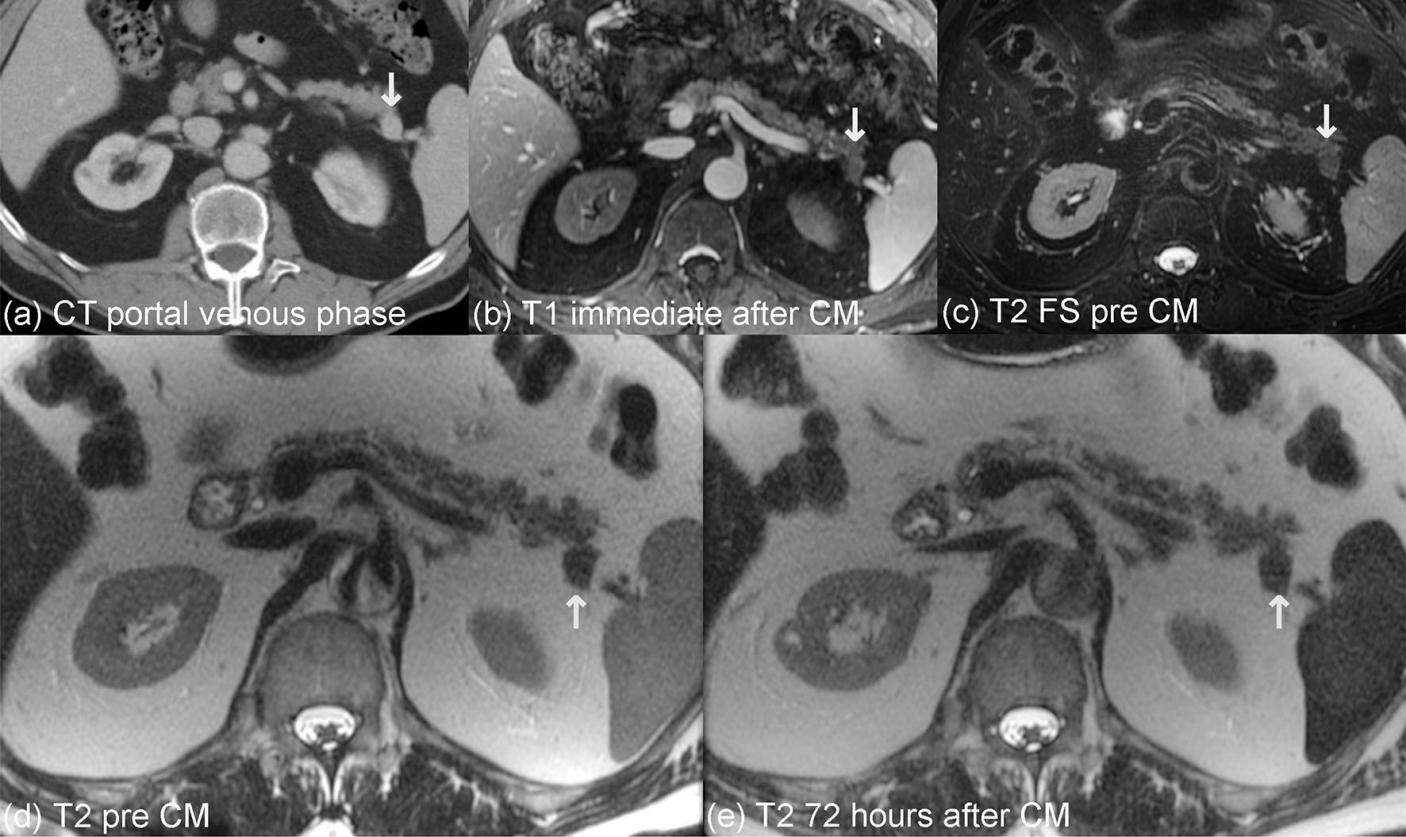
Imaging behavior of a pathologically proven PNET with feMRI (pt. 6). CT demonstrates an enhancing lesion (white arrow, a), which has relatively poor enhancement on T1w imaging immediately after administration of ferumoxytol in an intravascular phase at MRI #1, unlike the spleen (b). The PNET is hypointense to pancreas and spleen on T2w imaging (c, d) prior to contrast. 3 days after ferumoxytol administration, there is no change in the T2w signal of the lesion, unlike the liver and spleen which become relatively hypointense
